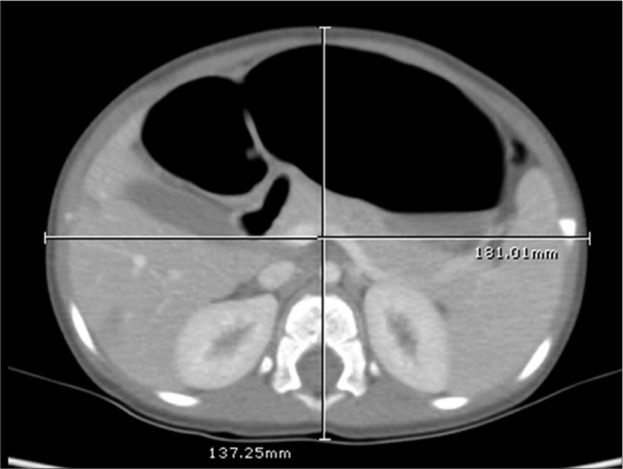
Contiguous chest and abdominal computed tomography (CT) demonstrating the anteroposterior and lateral dimension measurements. A 4-month-old boy weighed 6.1 kg underwent CT scan for tumor surveillance in underlying Langerhans cell histiocytosis.
